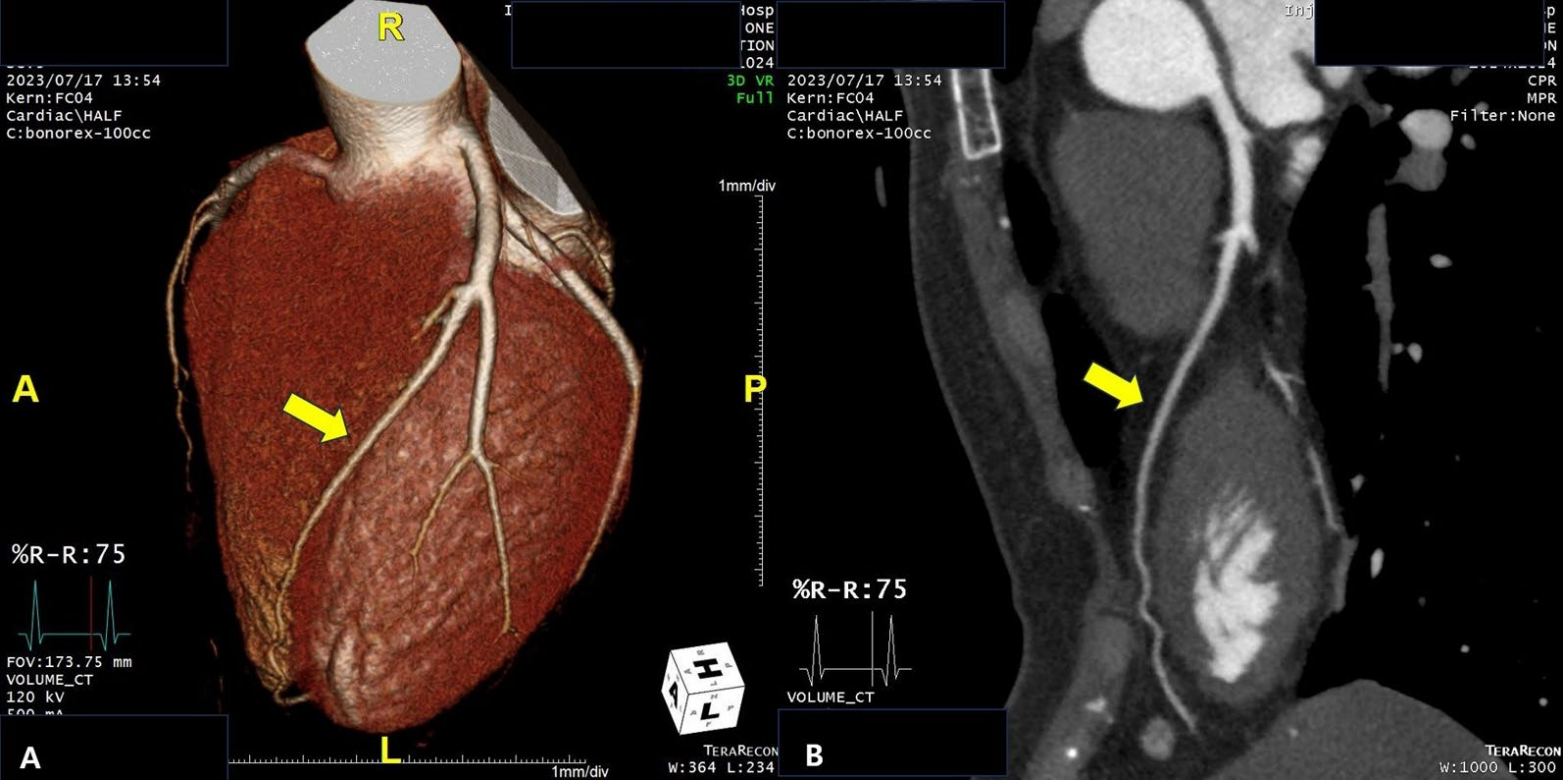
Follow-up coronary CT angiography [3D rendered view (A), 2D view (B)] of well healed LAD showing near normal coronary artery finding
